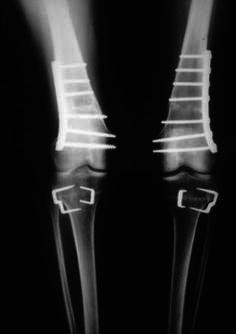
Patient DT. Radiograph after correction: supracondylar osteotomy of the femur fixed with plate and screws and proximal tibial osteotomy fixed with staples
